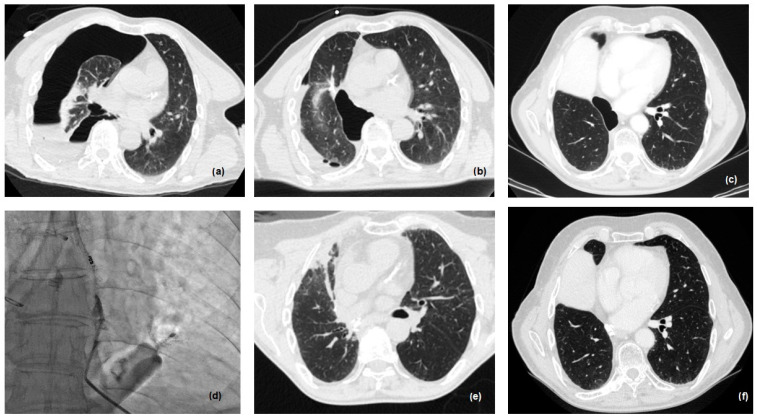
Percutaneous treatment of small and late BPF: (a) pneumothorax after inferior bilobectomy, 2 months after surgery; (b) drainage of pleural cavity; (c) persistence of pneumothorax after 3 months with fiberoptic diagnosis of BPF; (d) n-butyl cyanoacrylate (nBCA) glue injection under CT guidance, via 22-gauge Chiba needle into the postulated site of air leak/BPF; (e) immediate post-procedure CT scan control; (f) CT scan after 6 months.
